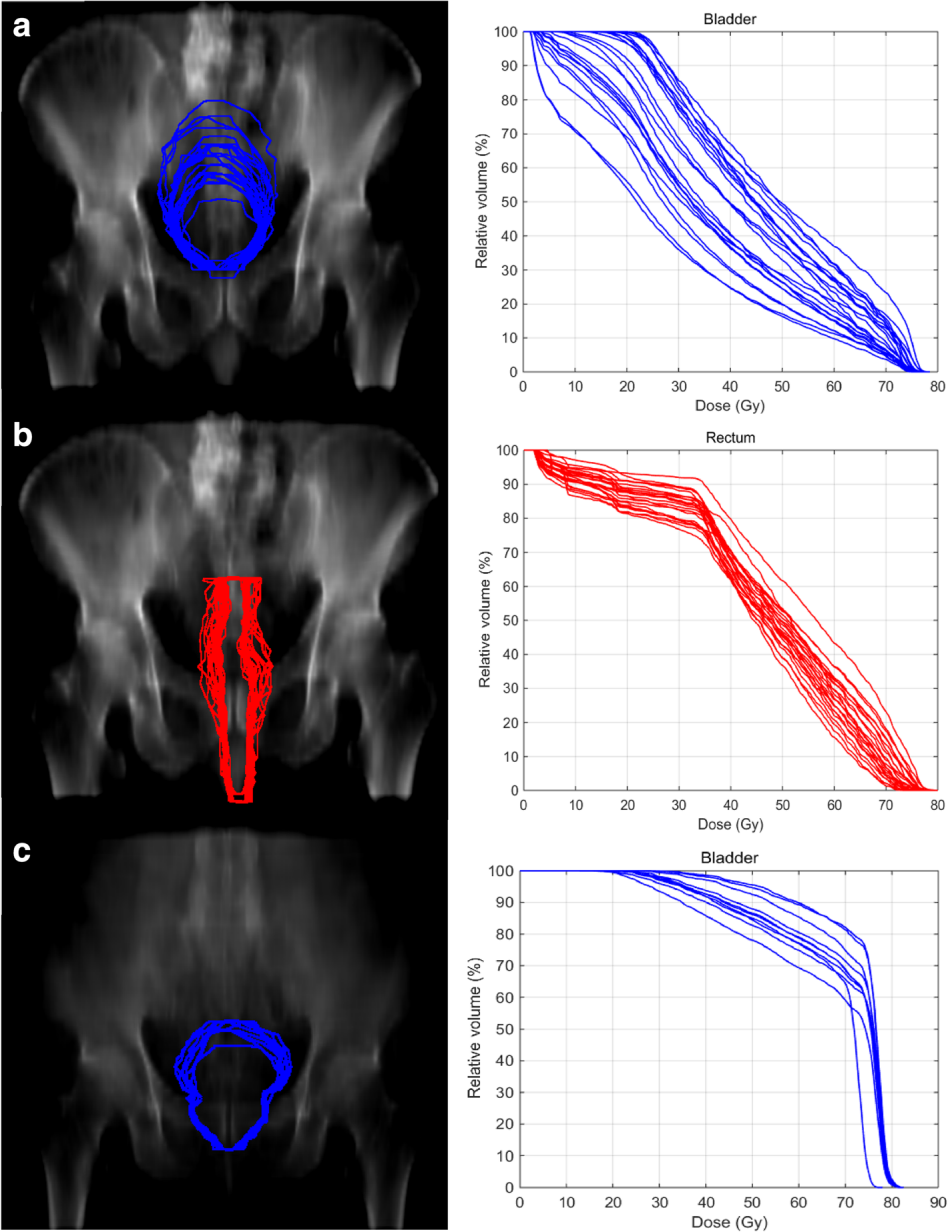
DRR of two patients. a–b show bladder and rectum contours for one patient derived from the different CBCTs projected on one DRR. The corresponded DVHs are displayed as well. c demonstrates different projections of the bladder contours on one DRR for a patient who had only very slight changed in his bladder filling
